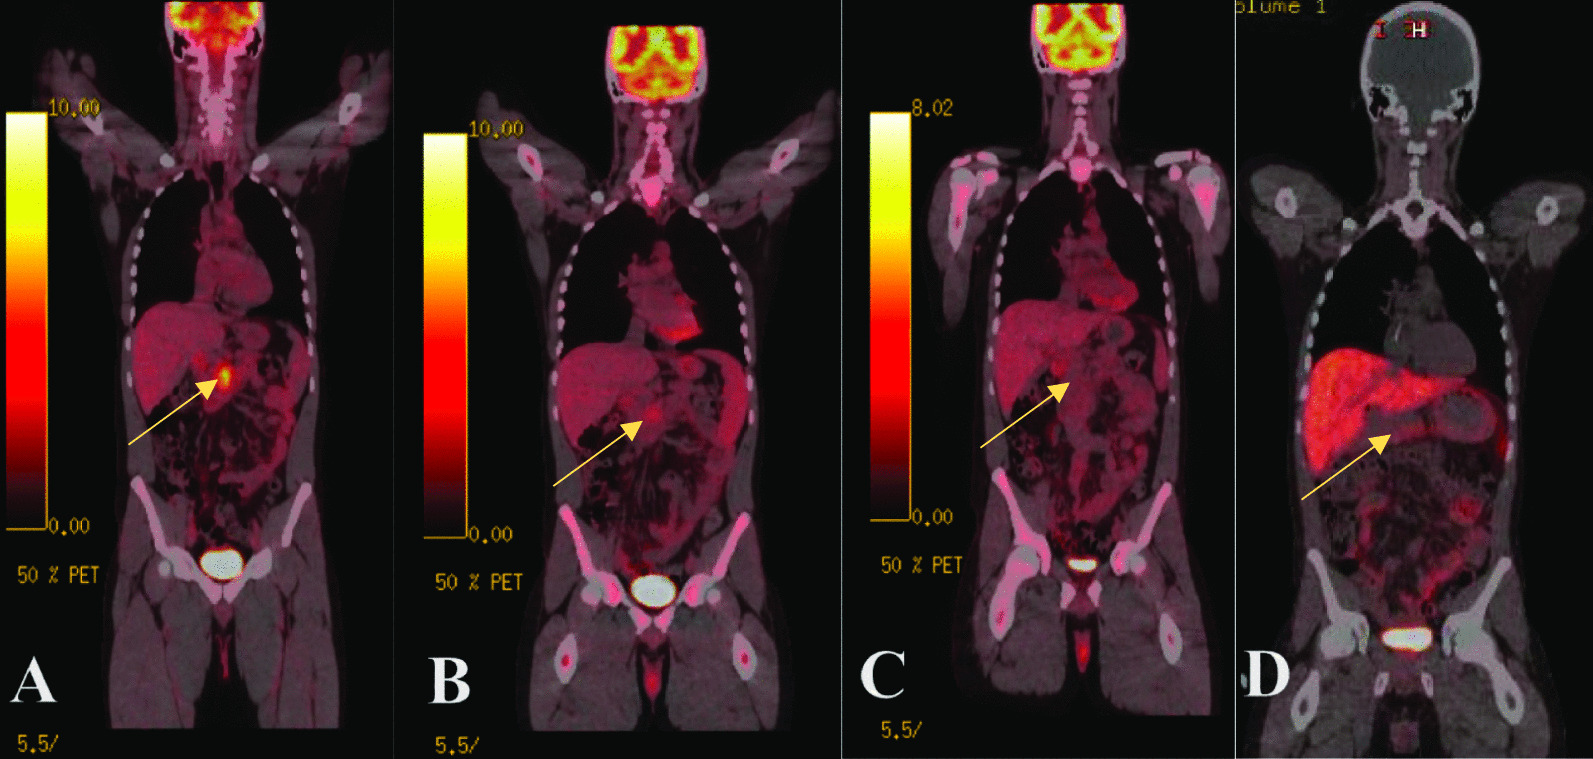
A PET scan prior to treatment (4/23/2019) showing moderate FDG avidity in the pancreatic mass, with an SUV max reaching 7.5 g/mL (Arrow). There is some uptake in adjacent nodes. B PET scan after 3 cycles (6/6/2019) of cisplatin/etoposide showing decrease in the PET activity involving the pancreatic mass with a maximum SUV value of 3.2 versus 7.6 on prior examination (Arrow). The adjacent peripancreatic PET avid lymph node also demonstrates decreased activity. C PET scan after 6 cycles (9/20/2019) of cisplatin/etoposide showing resolution of mild residual uptake in the peripancreatic foci (Arrow). D Post-operative PET Gallium without definite increased radiotracer uptake in the surgical bed to suggest DOTATATE-avid residual disease. Low level activity along the pancreatic remnant is nonspecific
